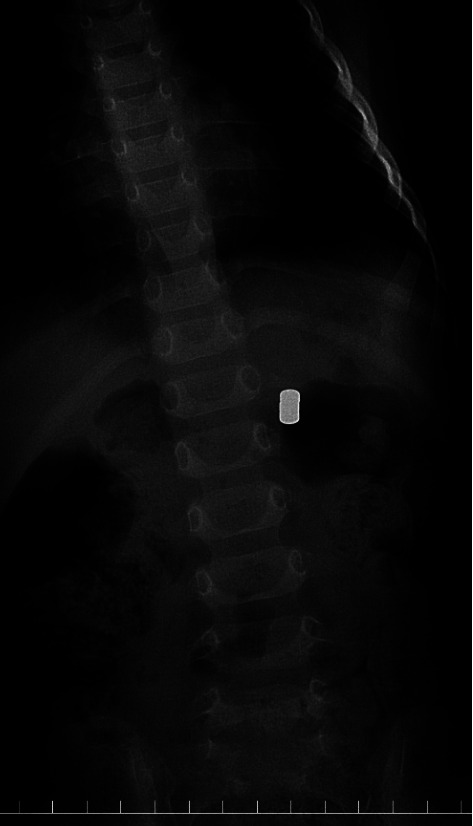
Abdominal radiography revealed a foreign body in the left upper quadrant, which were the three circular magnets.
